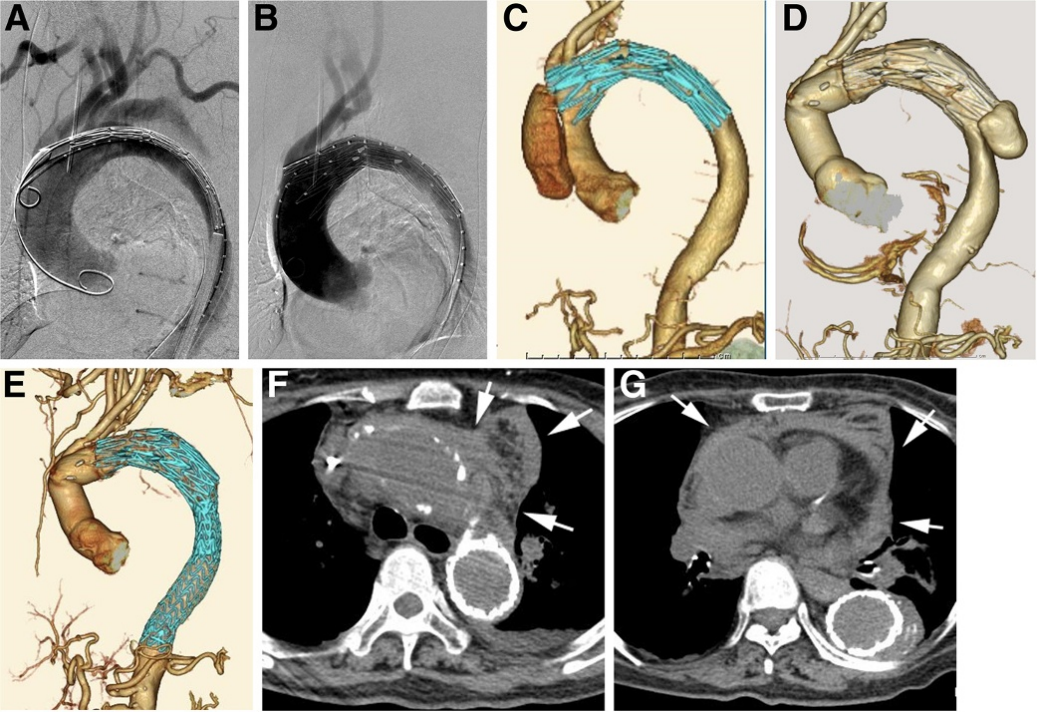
An 81-year-old female with type B chronic AD. A-C. One year after onset, oblique sagittal MPR images and aortography show aneurysmal dilation of the affected aorta. D. TEVAR (handmade stent-graft: 38-mm diameter & 20-cm length) was performed the entry site was closed. E. Three months after TEVAR, 3D CT angiography shows a type A AD due to a new intimal tear caused by the proximal edge of the stent-graft. Ascending aorta replacement was conducted. F. Three months after surgery, 3D CT angiography shows a type B AD due to a new intimal tear caused by the distal edge of the stent-graft. G. Re-TEVAR (GORE TAG: 37-mm diameter & 20-cm length × 2) was performed. 3D CT angiography images show that false lumen fully thrombosed.
